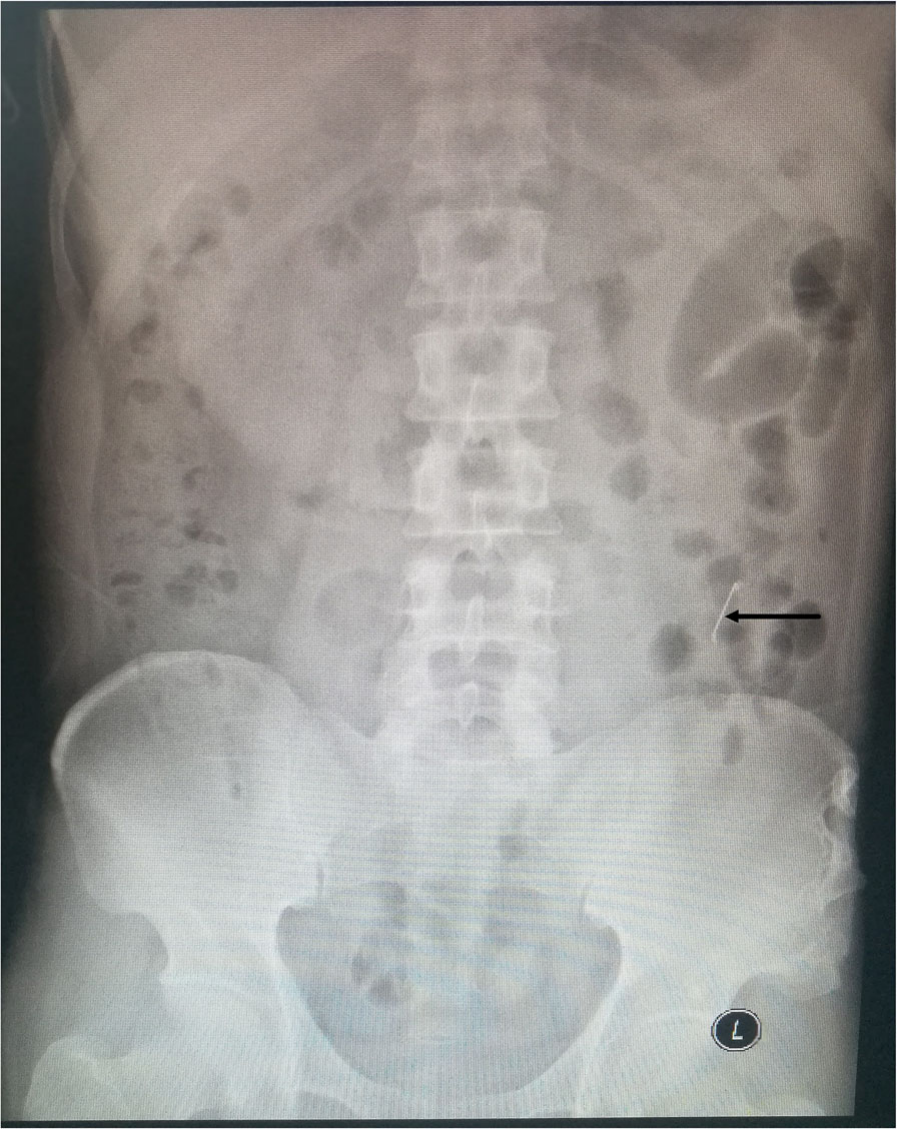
Preoperative Abdominal X-ray. The broken acupuncture needle was showed by arrow
